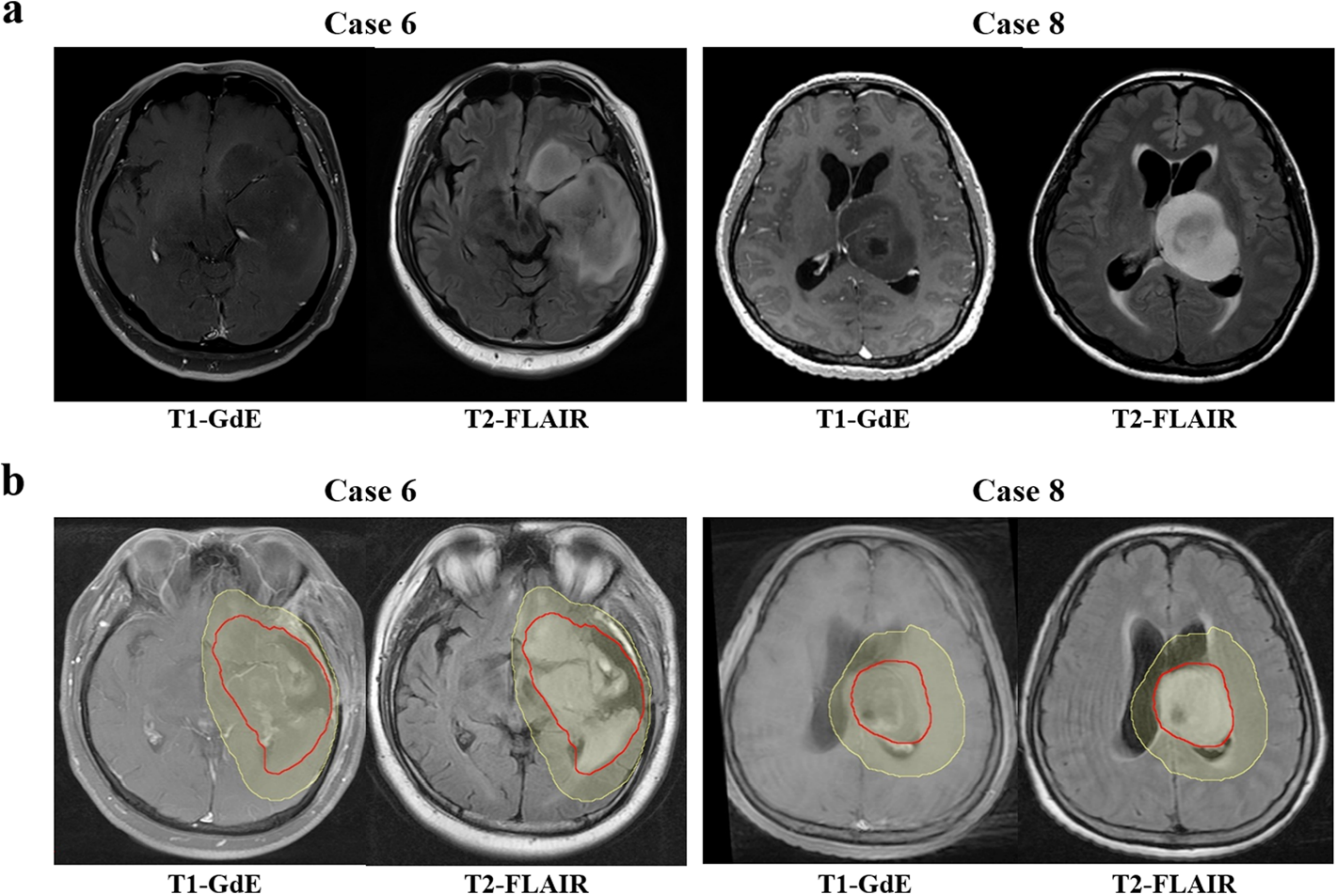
a Pre-operative/biopsy gadolinium enhanced T1-weighted (T1-GdE) and T2-FLAIR images of case 6 and 8. b GTV_STAPLES_ (red) and CTV_STAPLES_ (yellow) contoured on post-operative/biopsy MRIs of case 6 and 8
